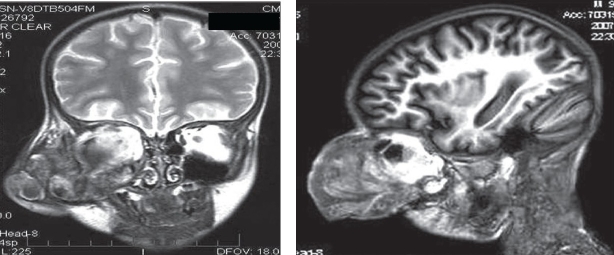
(T2 W-MRI images) Well-defined enhancing irregular mass and hyperintense periphery in the retrobulbar space
